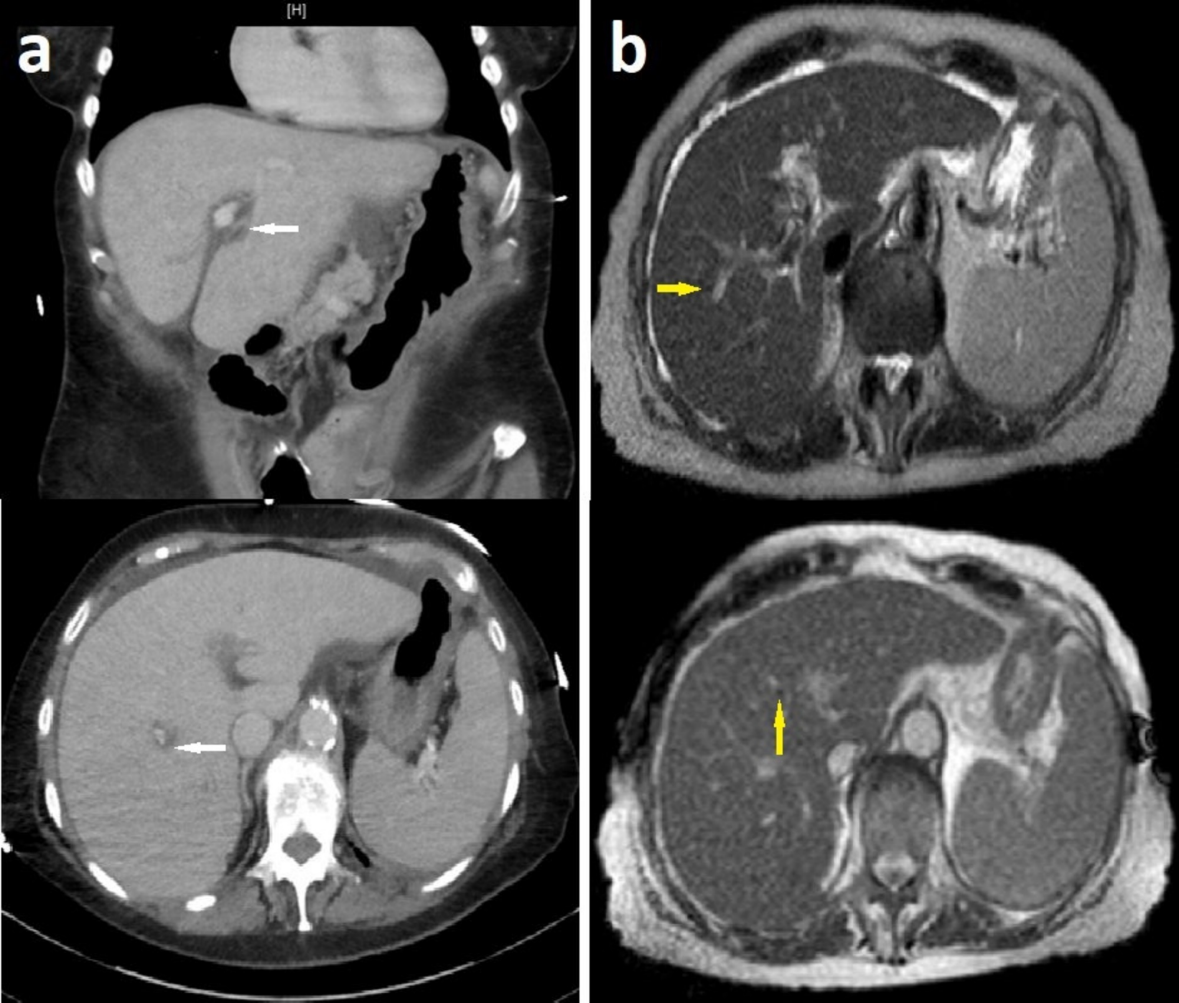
(a) Computed tomography of the liver (coronal and axial planes) showing periportal edema (white arrows); and (b) periportal halos (yellow arrows) on magnetic resonance imaging (T1 and T2-weighted images)
